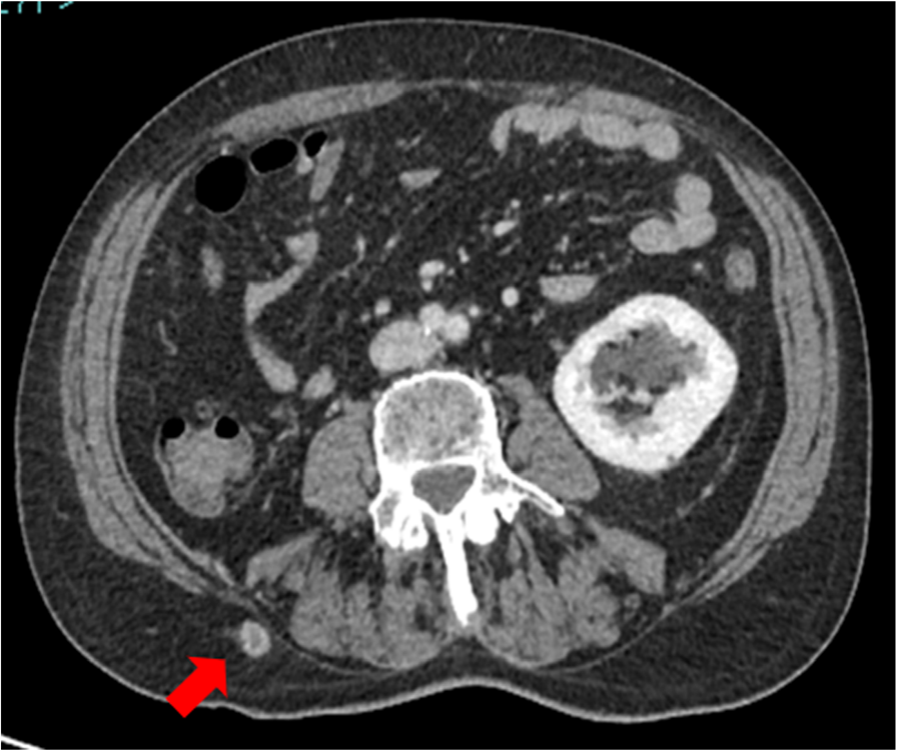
CT scan showed in the context of the subcutaneous soft tissues of the posterior abdominal wall, in the lumbar region, a nodule suspected for a subcutaneous metastasis
